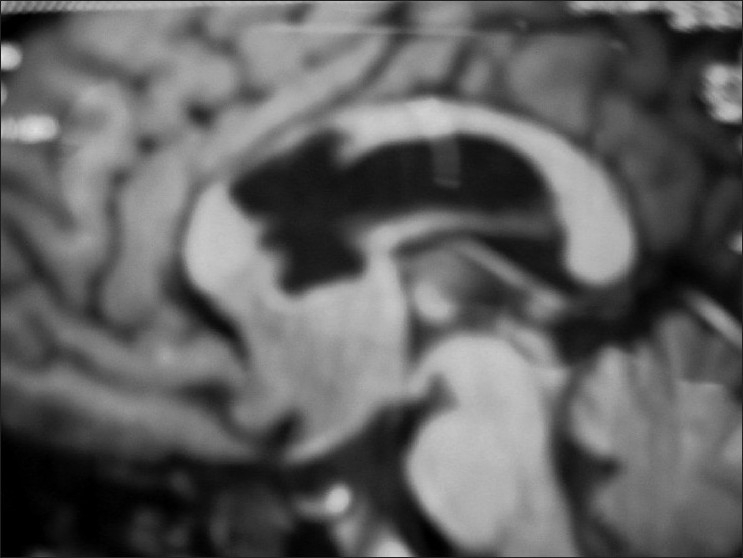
Post-operative magnetic resonance imaging showing the site and residual disease in the third ventricle
